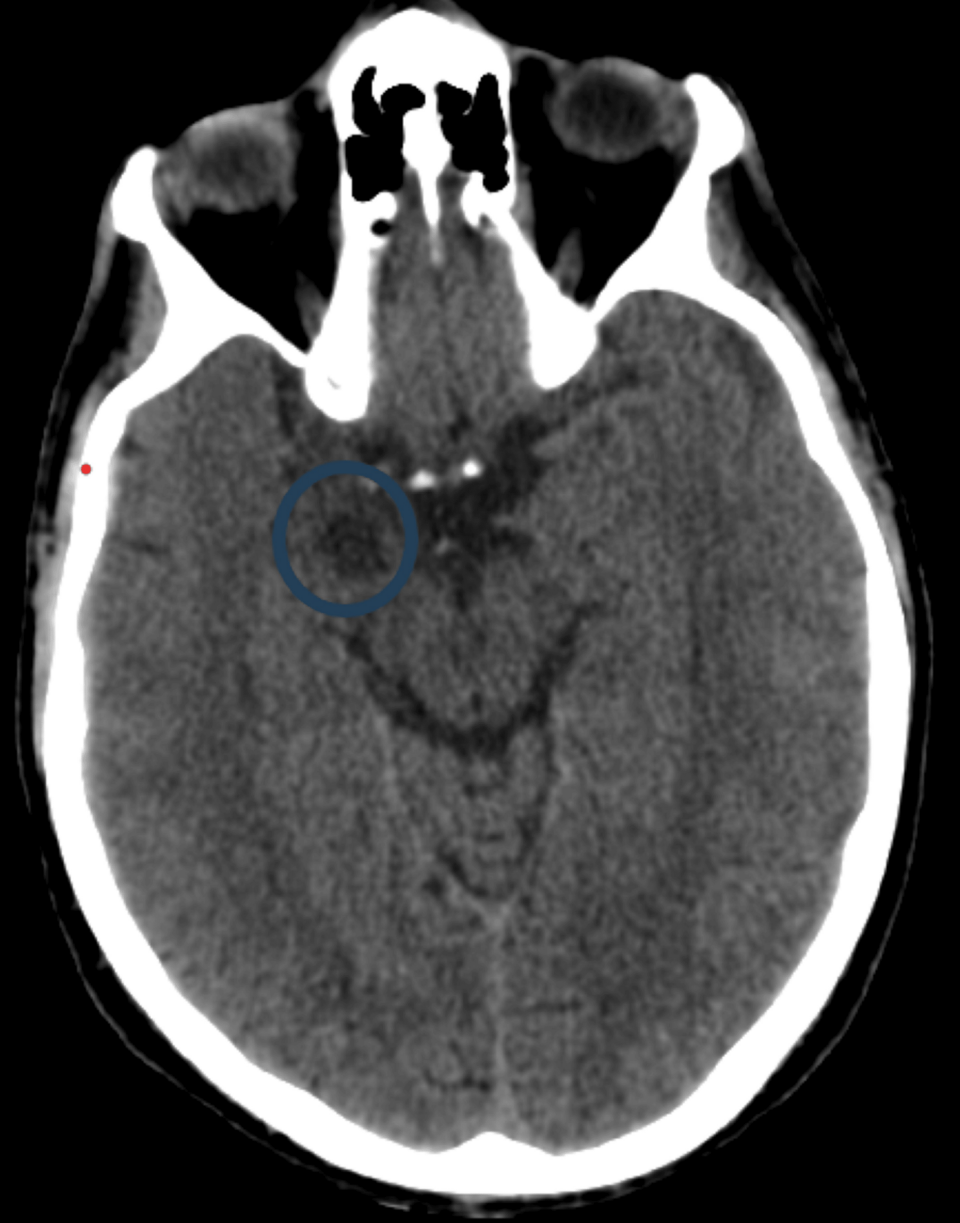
A CT of the brain, taken at the time of admission, revealed a hypodense lesion in the right hippocampus and amygdala (blue circle).
